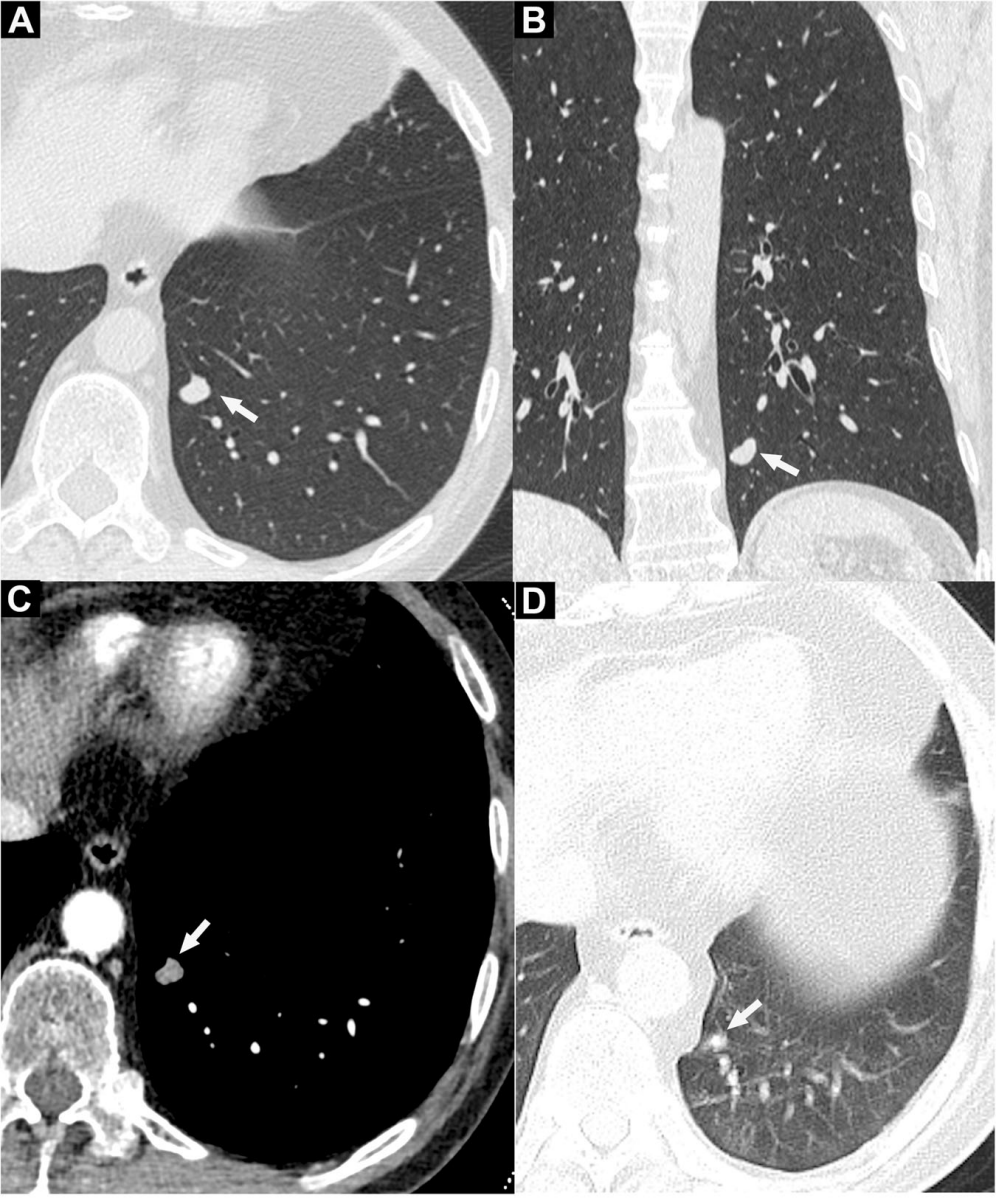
Follow-up CT scans to locate a possible ACTH-secreting tumor in a 48-year-old man with ectopic Cushing’s syndrome (A–D), The lung nodule was pathologically confirmed as typical carcinoid. No abnormality was found in the somatostatin receptor scintigraphy. Both ^18^F-FDG PET/CT and ^68^Ga-DOTATATE PET/CT did not show increased uptake in the small nodule in the left lower lobe (LLL). Chest CT showing a small peripheral solid nodule (diameter 0.9 cm) in the LLL (arrows in A–C). A Axial and (B) coronal 1 mm thin-slice non-contrast CT images at the lung window showing a nodule with a clear boundary and lobulated shape. C Thin-slice axial contrast-enhanced CT at the mediastinal window showing moderate homogeneous enhancement (arrow, Δ CT value = 54 HU after contrast injection). D The micro solid nodule (diameter 0.4 cm) in the LLL (arrow) was misdiagnosed as a cross-section of a blood vessel on the baseline CT five years prior. The misdiagnosis may have been caused by hindsight bias, poor image quality influenced by a slight respiratory motion artifact, and the 5 mm thick-slice thickness
